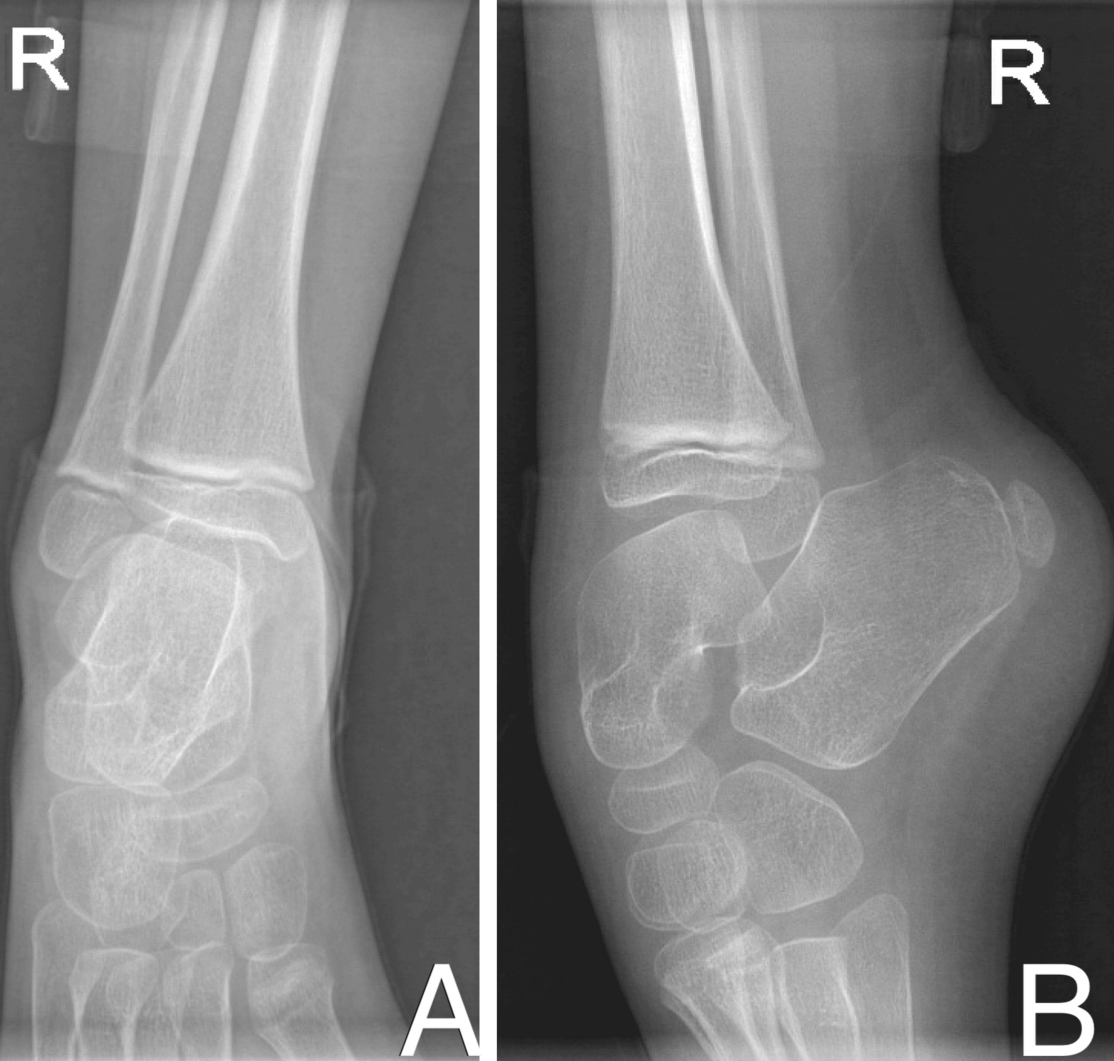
Preoperative X-ray of the right side Preoperative anterior-posterior (A) and lateral (B) X-ray of right ankle showing equinus deformity without obvious bony abnormality.
